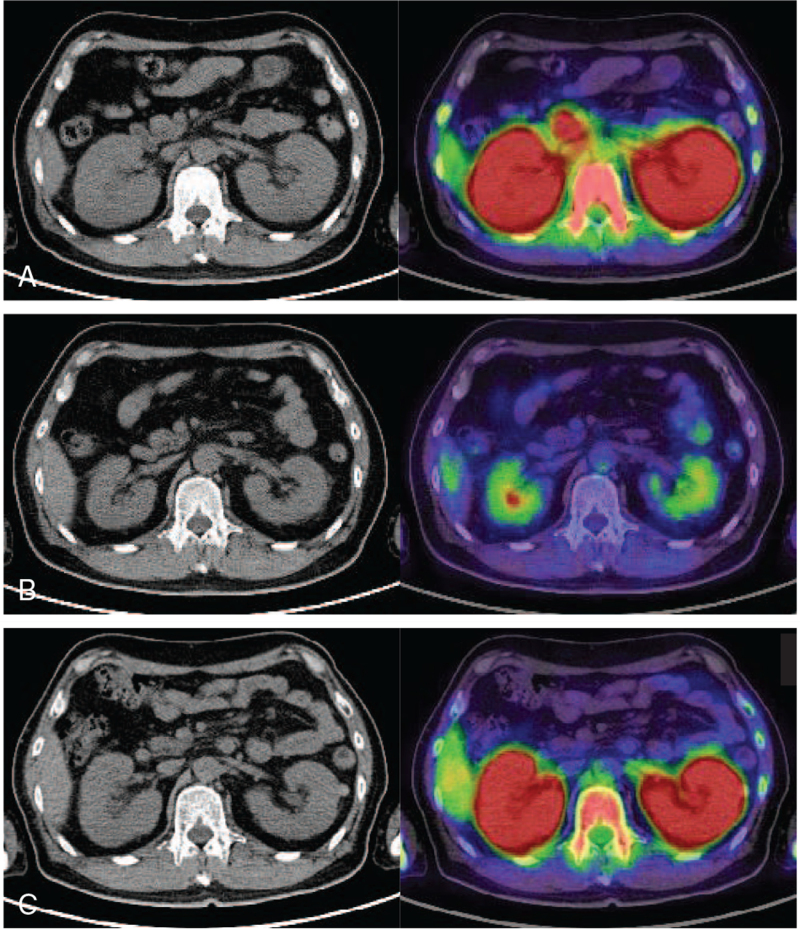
Pre-and posttreatment 18-fluorodeoxyglucose (^18^FDG) positron emission tomography (PET)-computed tomography (CT) of the abdomen (A) ^18^FDG PET/CT at diagnosis showing ^18^FDG-strong uptake in bilateral enlarged kidneys. (B) ^18^FDG PET/CT posttreatment showing no abnormal ^18^FDG uptake in the bilateral kidneys. (C) ^18^FDG PET/CT at relapse showing ^18^FDG-strong reuptake in bilateral enlarged kidneys.
